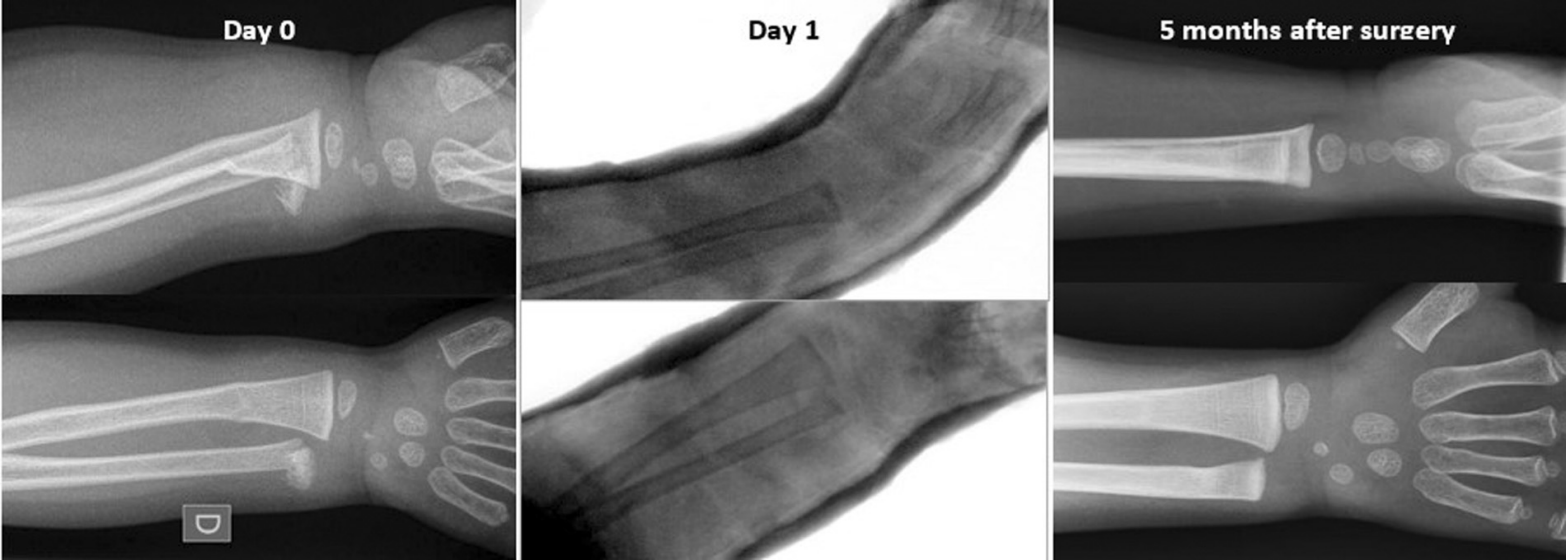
Serial X-rays of the patient.
